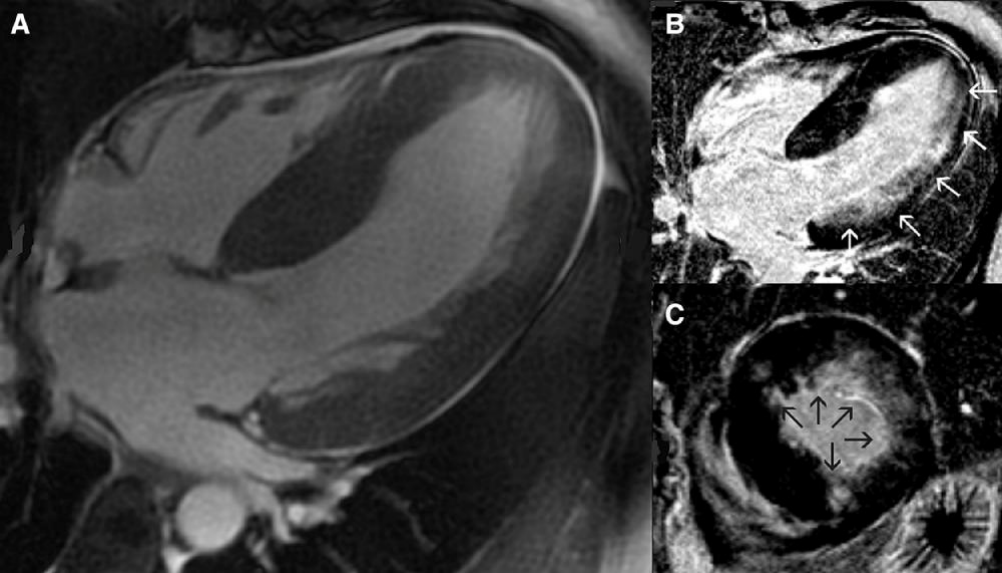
Magnetic resonance imaging depicting: (*A*) significant hypertrophy of the left ventricular walls. (*B*, *C*) Late gadolinium enhancement within the left ventricular wall (arrows).
